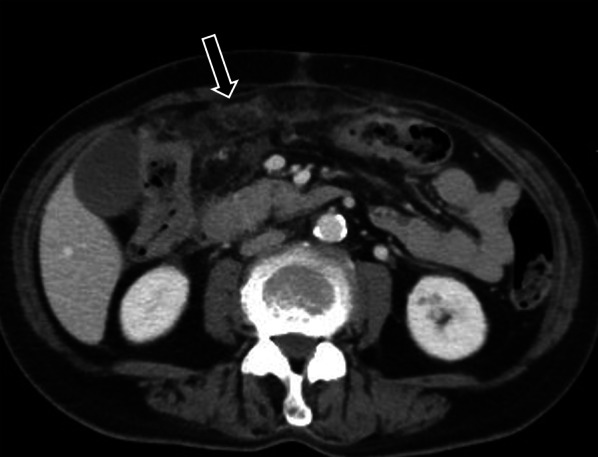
Metastatic peritoneal disease on staging CT, in a 61-year-old female with invasive lobular carcinoma. Axial contrast-enhanced CT image shows multiple, ill-defined, tiny nodules in the peritoneum (arrow), which were highly suspicious for metastatic disease. This was confirmed on diagnostic laparoscopy and biopsy
